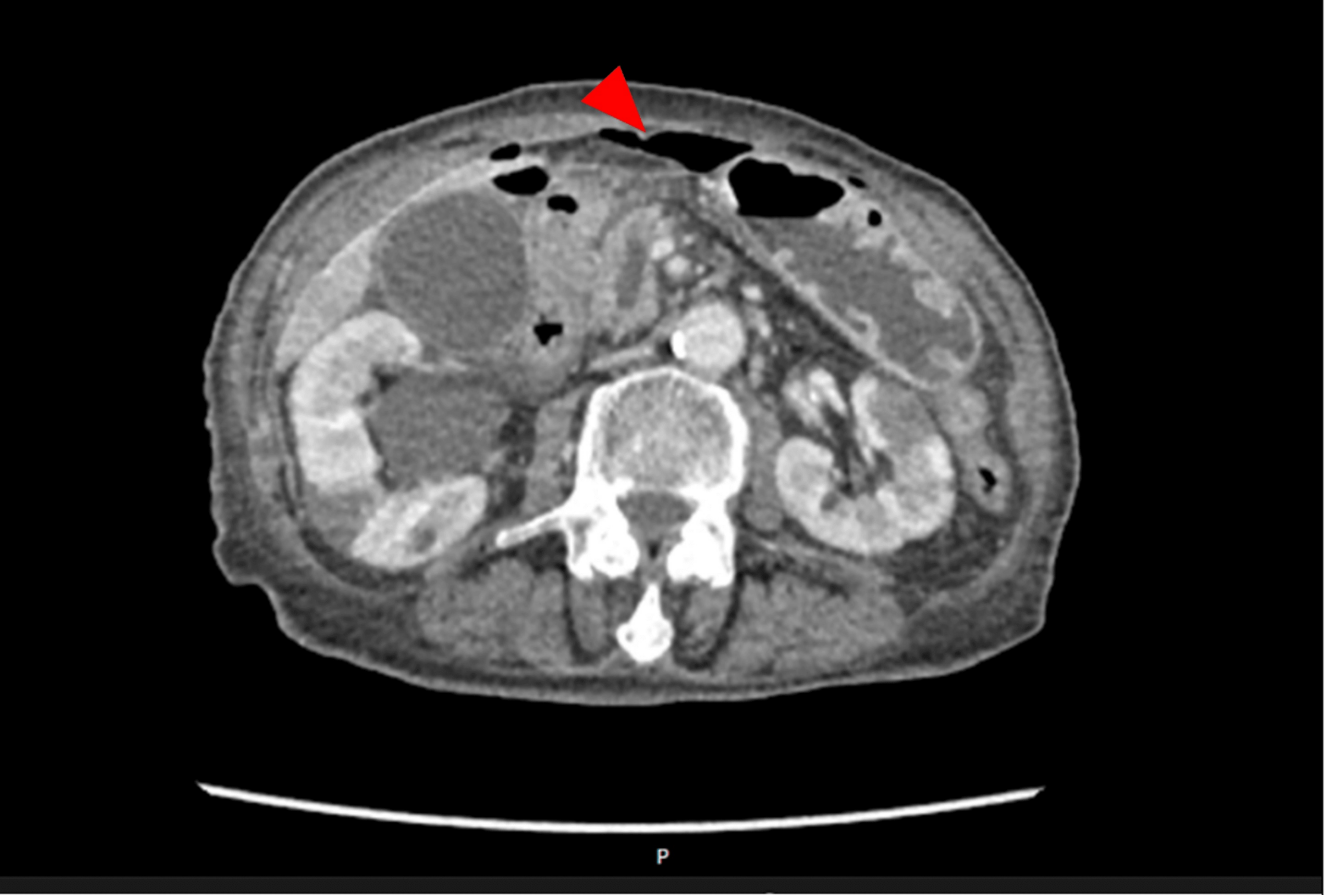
CT of the abdomen and pelvis showing free subdiaphragmatic gas (red arrow).
